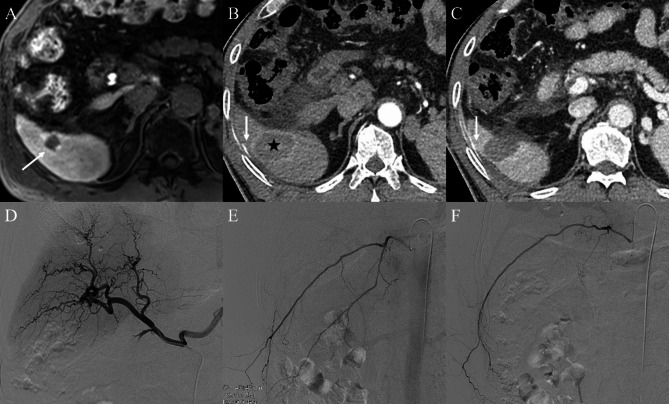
A case of a 66-year-old male showing contrast extravasation on post-ablation computed tomography (CT) following radiofrequency ablation (RFA). (A) Pre-procedure liver dynamic magnetic resonance imaging (MRI) revealed hepatocellular carcinoma (HCC) in the segment VI subcapsular area (arrow). (B, C) After completion of ablation, post-ablation three-phase dynamic contrast-enhanced CT was performed. The tumor was completely included in the ablation zone (asterisk); however, contrast extravasation was observed (arrows). (D, E, F) Nevertheless, no evidence of active bleeding was observed on transarterial angiography, including hepatic and intercostal arteriograms
